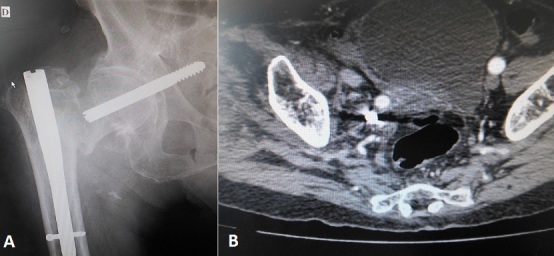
A) radiographie du bassin de face montrant la migration de la vis cervicale en intra pelvien; B) angioscanner pelvien montrant la proximité de la tête de vis des vaisseaux iliaques
